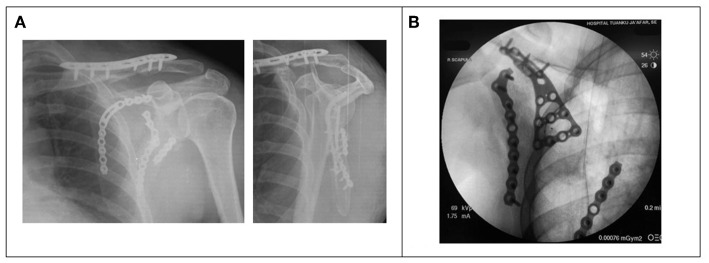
(A) Post-operative radiograph (AP and Y-view) showing clavicle plate, with medial and lateral locking plate *in situ*. (B) Fluoroscopy image of the Buttress plate used to press down “floating” segment of scapular fracture.
